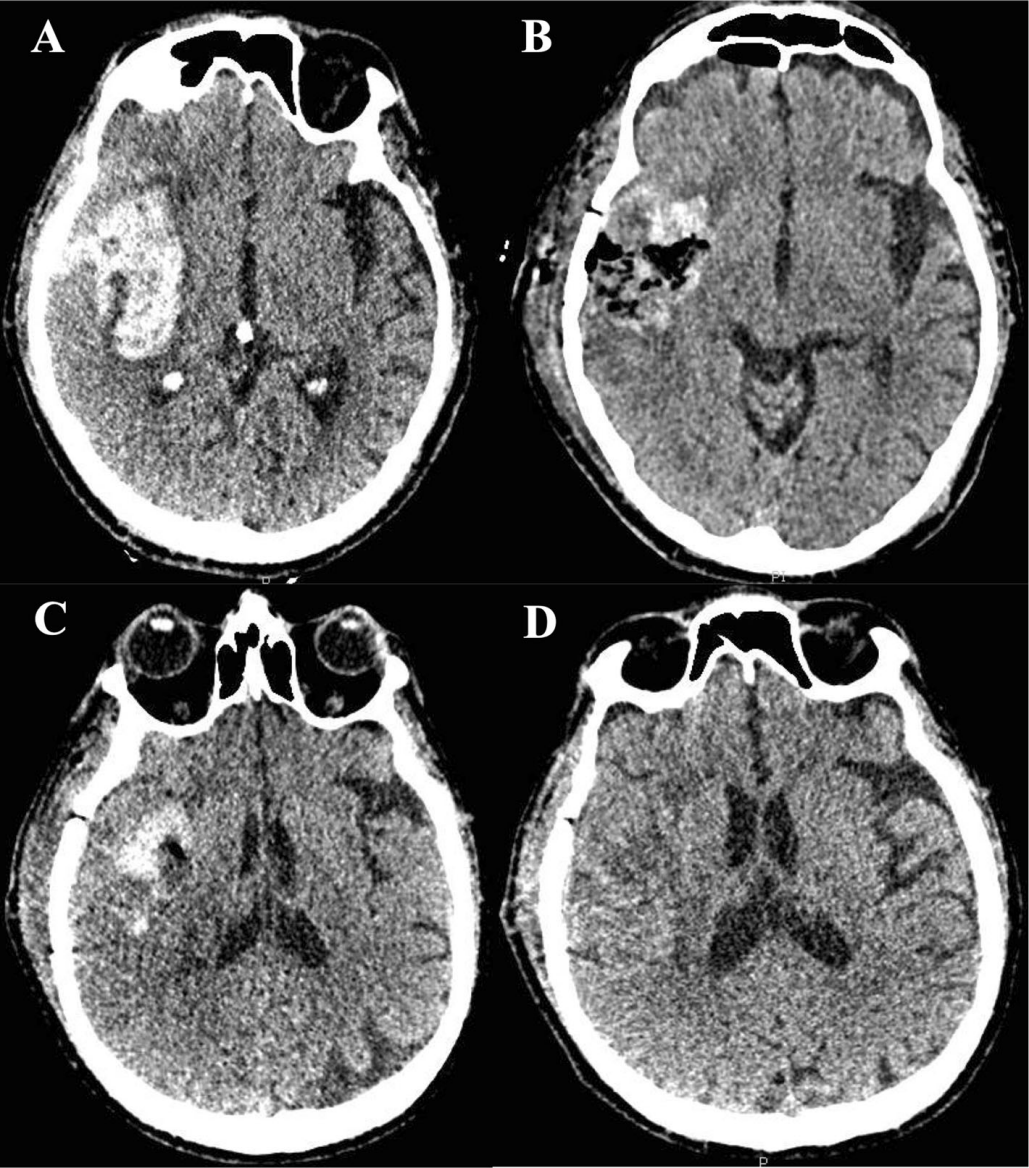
Case 01 of open craniotomy for hematoma drainage. a Day 1—a large intraparenchymal hematoma centered on the putamen, right insular, and frontotemporal region, with extravasation into the subarachnoid space of the sylvian fissure and temporal fossa, measuring about 6.1 × 4.5 × 4.8 cm on its largest axes. b Day 2—Hematoma was surgically removed by open craniotomy. CT shows signs of surgical manipulation characterized by enlargement and densification of soft tissue planes with gaseous foci underlying the right parietotemporal craniotomy. There was reduction of the dimensions of the intraparenchymal hematoma. c Day 7—Follow-up CT scan 6 days after surgical drainage. d Day 21—Follow-up CT scan 21 days after surgical drainage. Patient was discharged home after this last CT scan with a modified Rankin scale 4 (able to walk with assistance)
